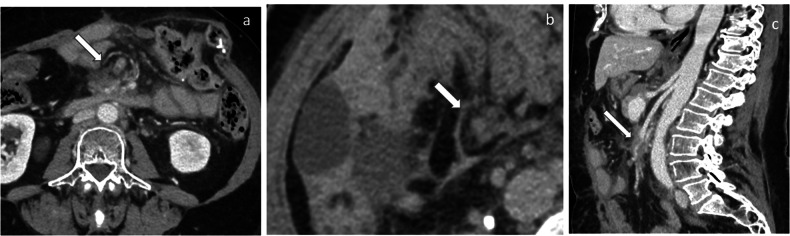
Small bowel mesentery Infiltration: axial (a, b) and sagittal (c) CT images of a diffuse deep infiltration (white arrows)
